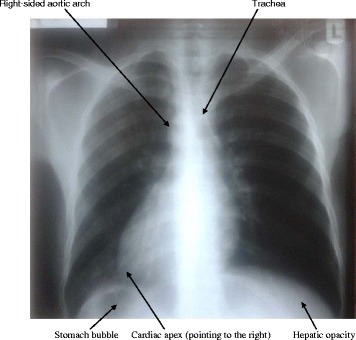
Chest X-ray (posterior anterior view) of a 22-year-old Nigerian man showing dextrocardia, with the cardiac apex pointing to the right. His aortic arch was located on the right. His stomach bubble was located below his right hemidiaphragm. His hepatic opacity was located below his left hemidiaphragm. His trachea was slightly deviated to the left
